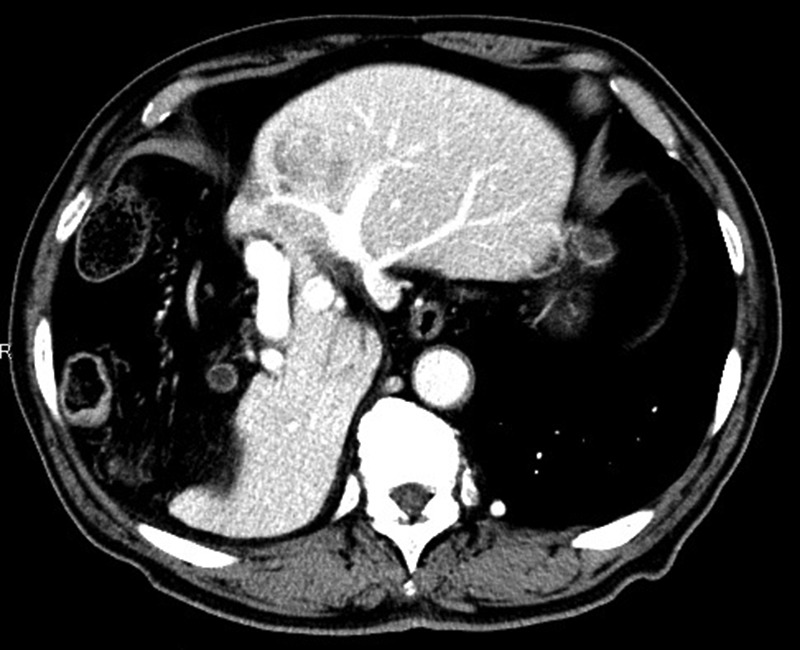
Dynamic contrast-enhanced CT showing a 40-mm nodule in S3 and hypoplasia of the right hepatic lobe with the Chilaiditi sign.
